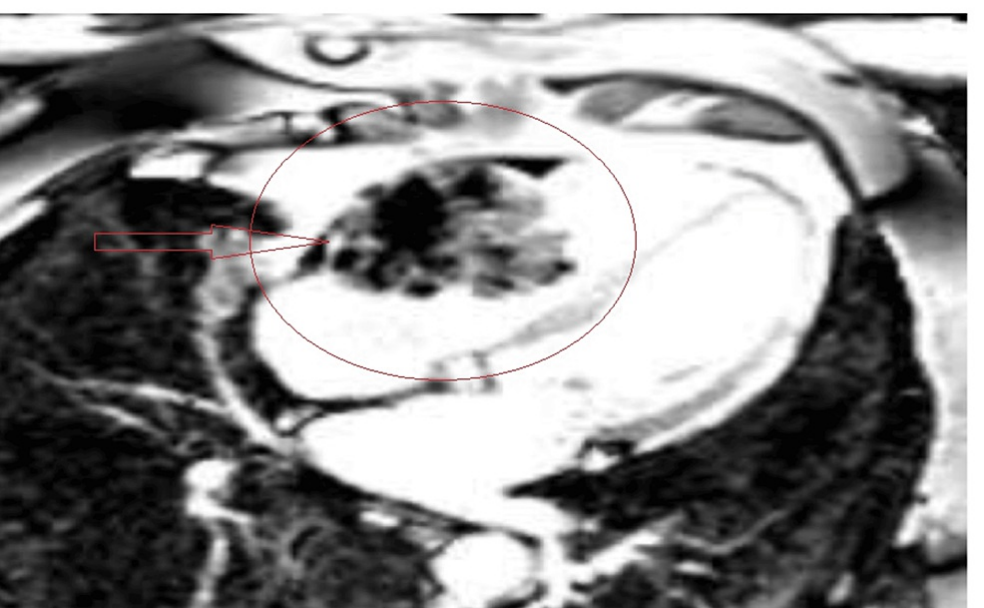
CT with contrast showed a 3 cm irregular mass in the right middle lobe, abutting the pericardium, several subcentimeter nodules, and no lymphadenopathy.
